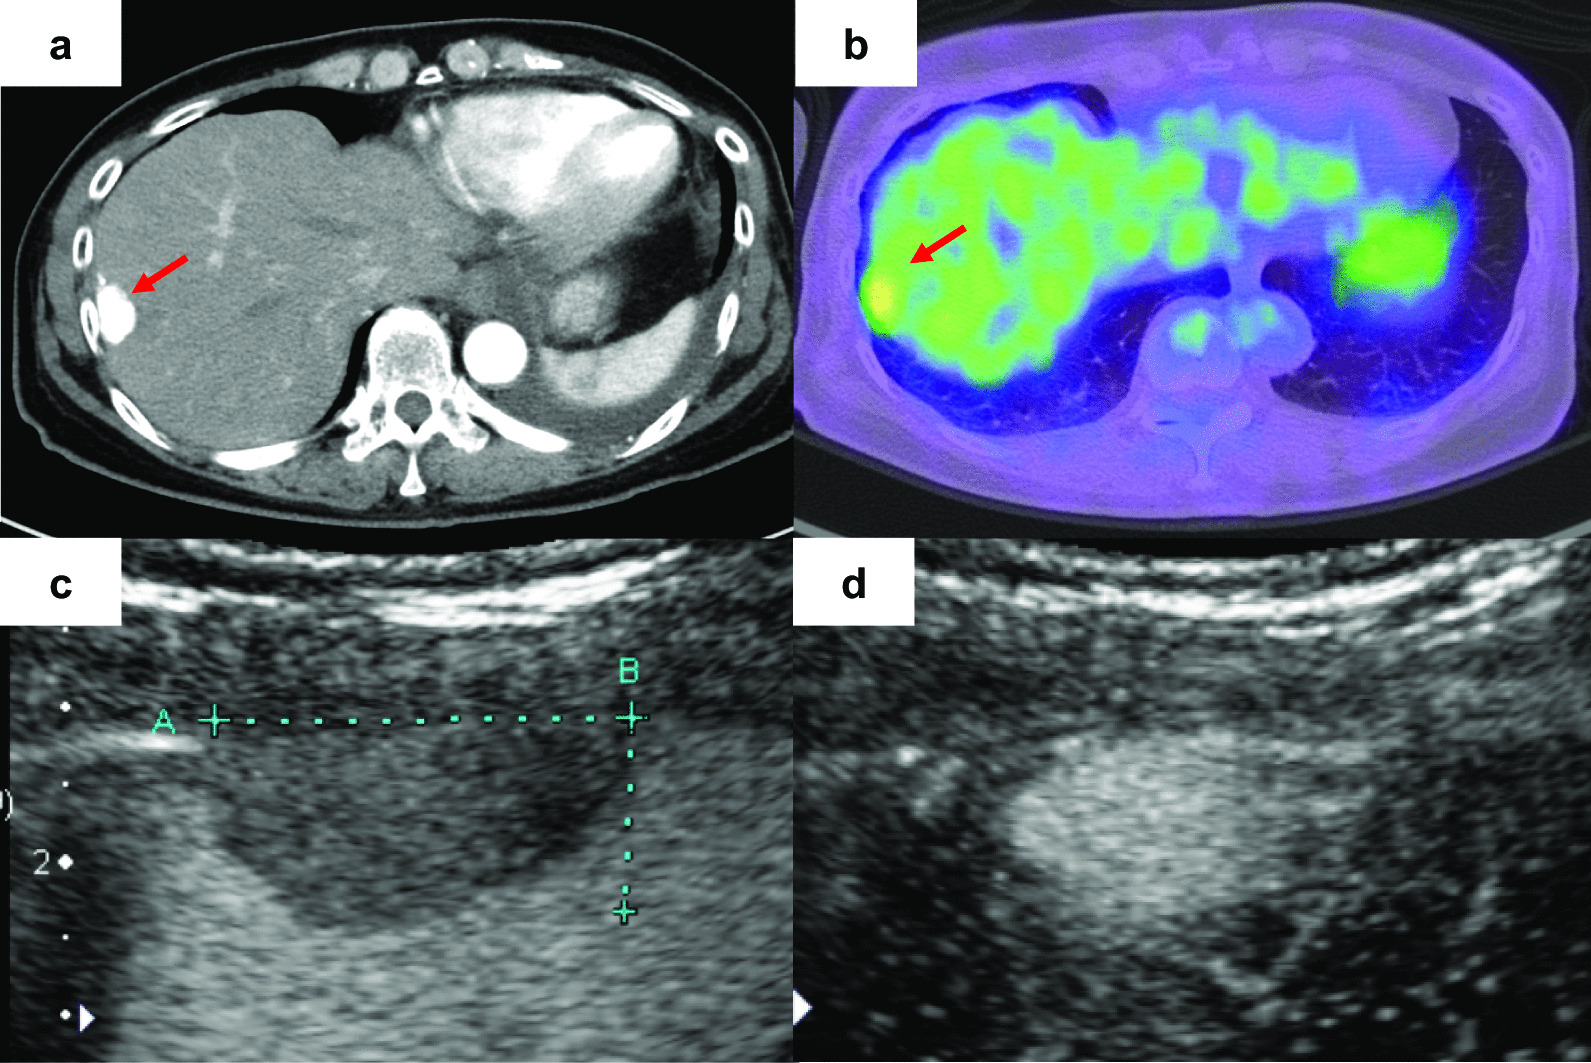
Preoperative examination. a Contrast-enhanced computed tomography revealed a tumor (arrow) with marked enhancement in the arterial phase of the right diaphragm. b 18-Fluoro-2-deoxyglucose positron emission tomography image revealed that the maximal standard uptake value of nodule (arrow) was 3.5. c Ultrasonography showed a low-echoic lesion. d Early enhancement was observed in Sonazoid-enhanced ultrasonography
